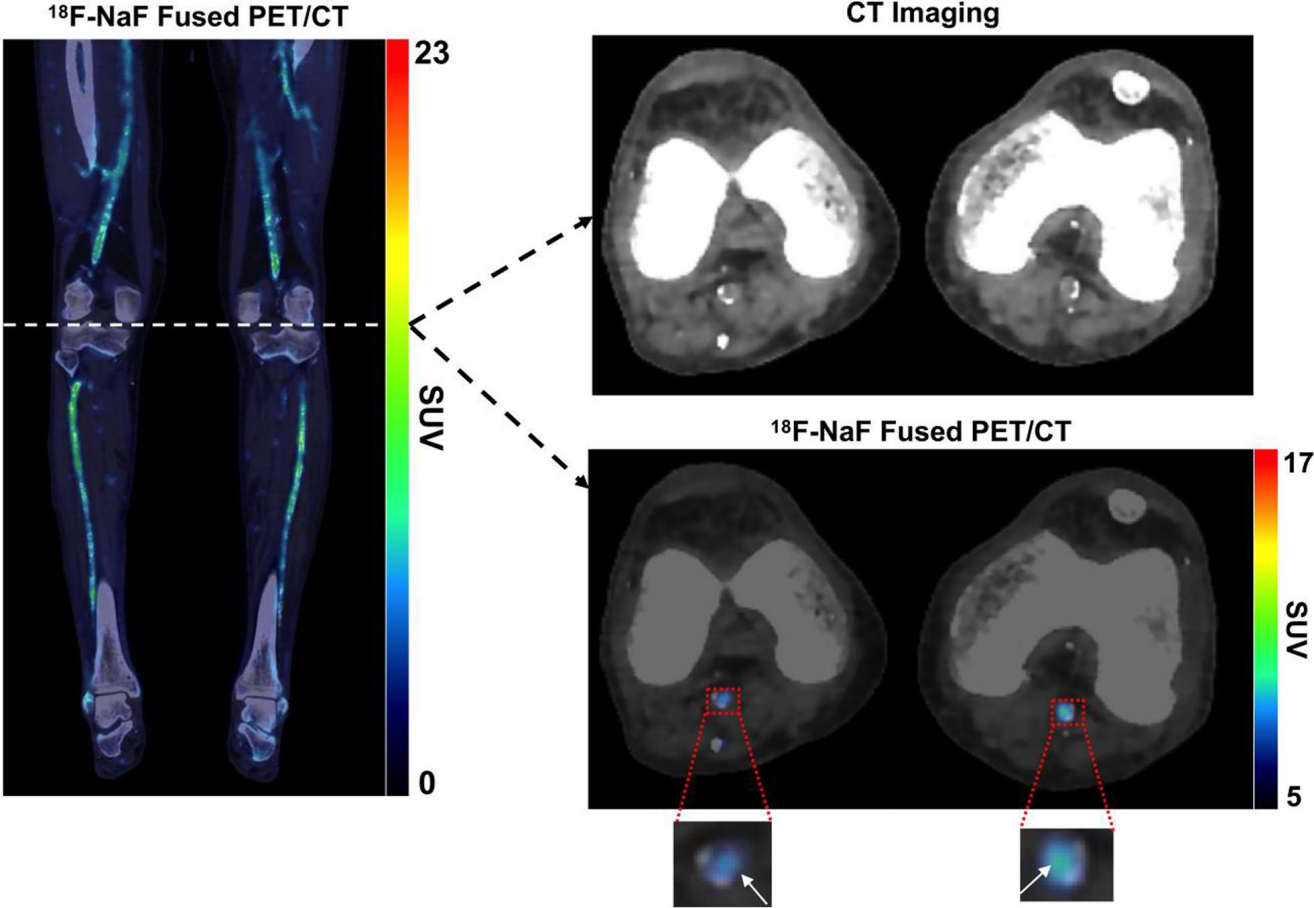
Representative ^18^F-NaF PET/CT imaging of the lower extremities in a patient with PAD and DM. Fused coronal ^18^F-NaF PET/CT imaging of both lower extremities demonstrated heterogenous macrocalcification detected by CT imaging and heterogenous arterial uptake of ^18^F-NaF detected by PET. Focused axial images at the level of the knee further revealed that increased ^18^F-NaF uptake was localized to arterial regions undergoing active microcalcification and were not yet fully detectable by CT imaging (denoted by white arrows)
